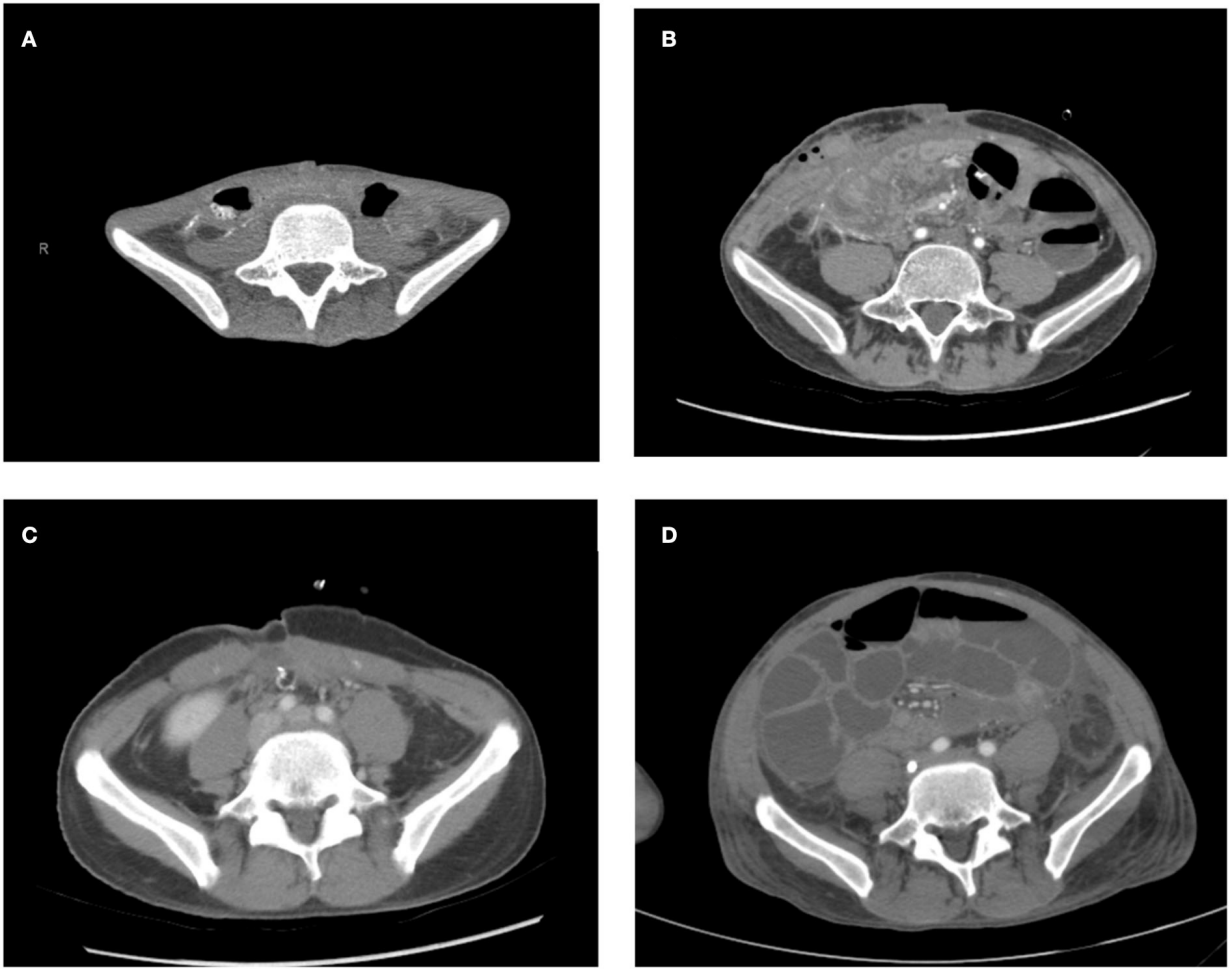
(A,C) CT before IT. (B,D) CT after IT, showing a significant increase of the abdominal area.
